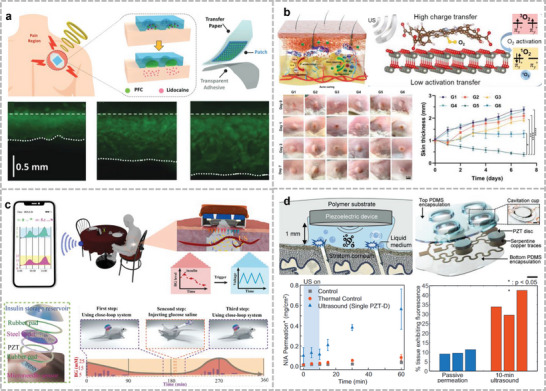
Ultrasound–assisted drug delivery. a) Non‐invasive topical drug delivery by acoustic droplet vaporization (ADV), which delivers the drug to the dermis and adjusts the amount of penetration by adjusting the applied ADV force. Reproduced with permission.^[^
